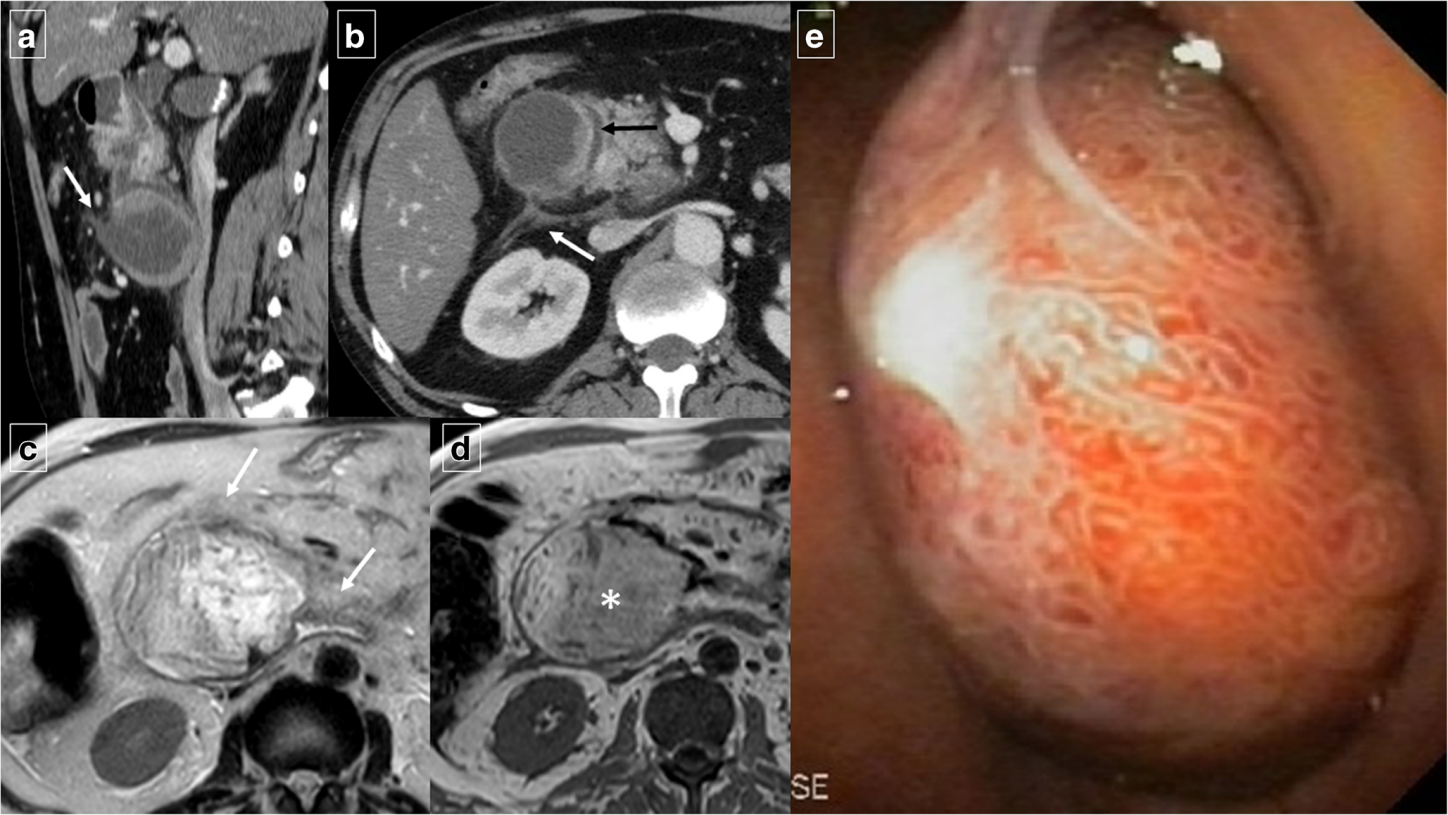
Ruptured duodenal duplication cyst in a 58-year-old man who sustained blunt trauma in a fall down stairs. a Reformatted sagittal contrast-enhanced CT shows circumferential wall thickening of the cyst and a disruption in its anterior surface (arrow). b Axial CT shows retroperitoneal fluid in the right anterior pararenal space (white arrow). The duodenal lumen is narrowed and displaced medially due to inflammatory changes (black arrow). c Axial T2-weighted MRI shows stranding of the periduodenal fat on (arrows). d Axial T1-weighted MRI image shows a hyperintensity of the content of the lesion (*). The high signal intensity (more than muscle tissue), in a traumatic context, suggests an haemorrhagic transformation of the cyst. e Detail of the fiberoptic endoscopic study
